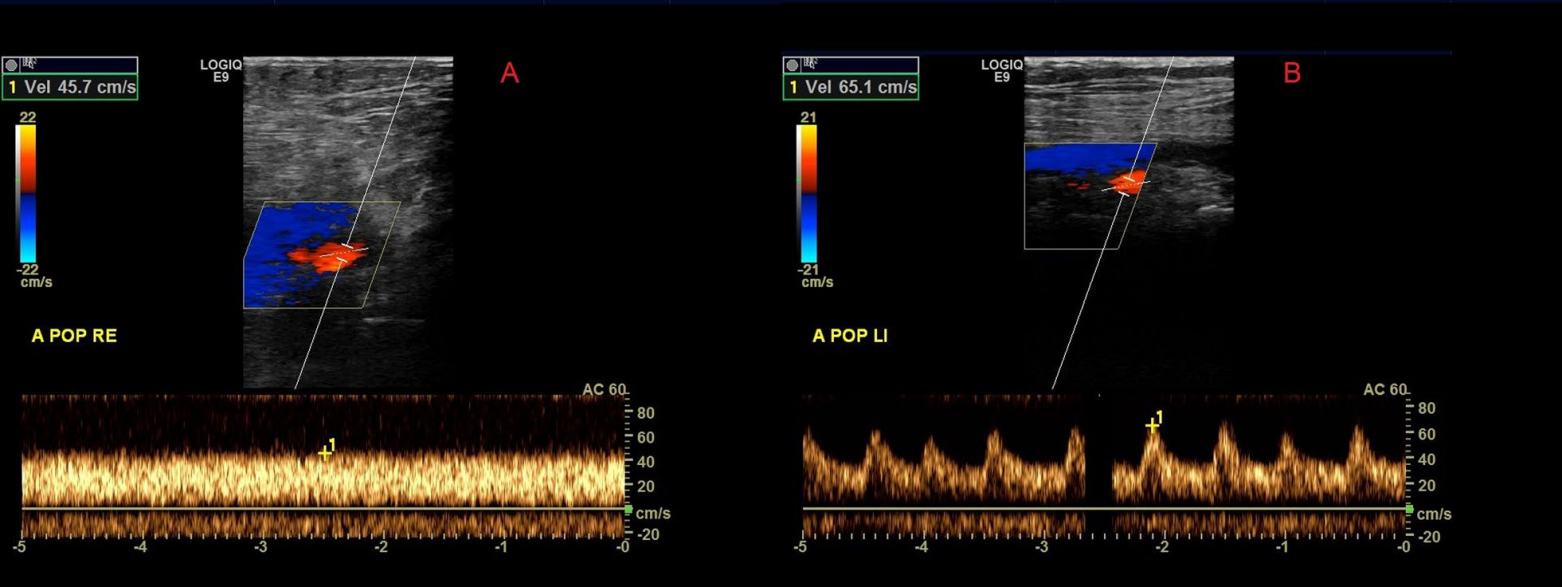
Color-coded duplex ultrasonography images of the leg with an arterial cannula (A) and the cannula-free leg (B)
